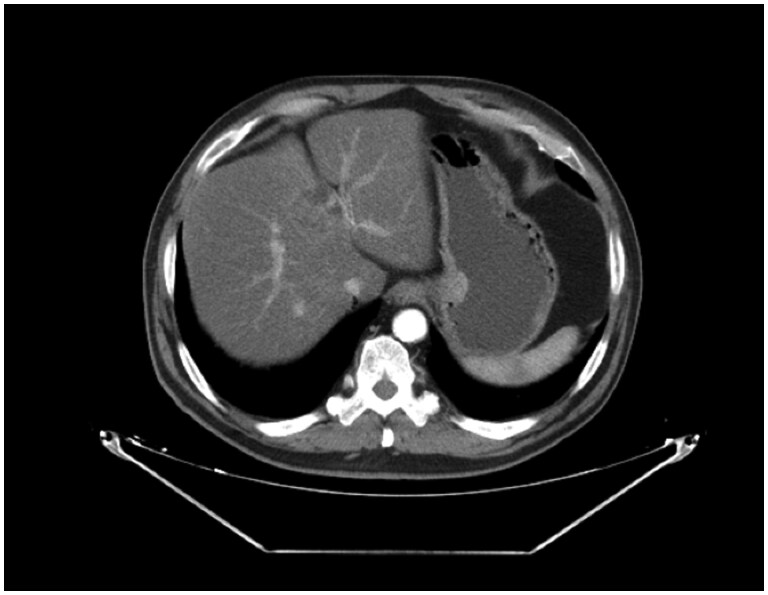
Axial contrast-enhanced CT-scan slice showing a well‑circumscribed submucosal mass on the lesser curvature of the stomach.
